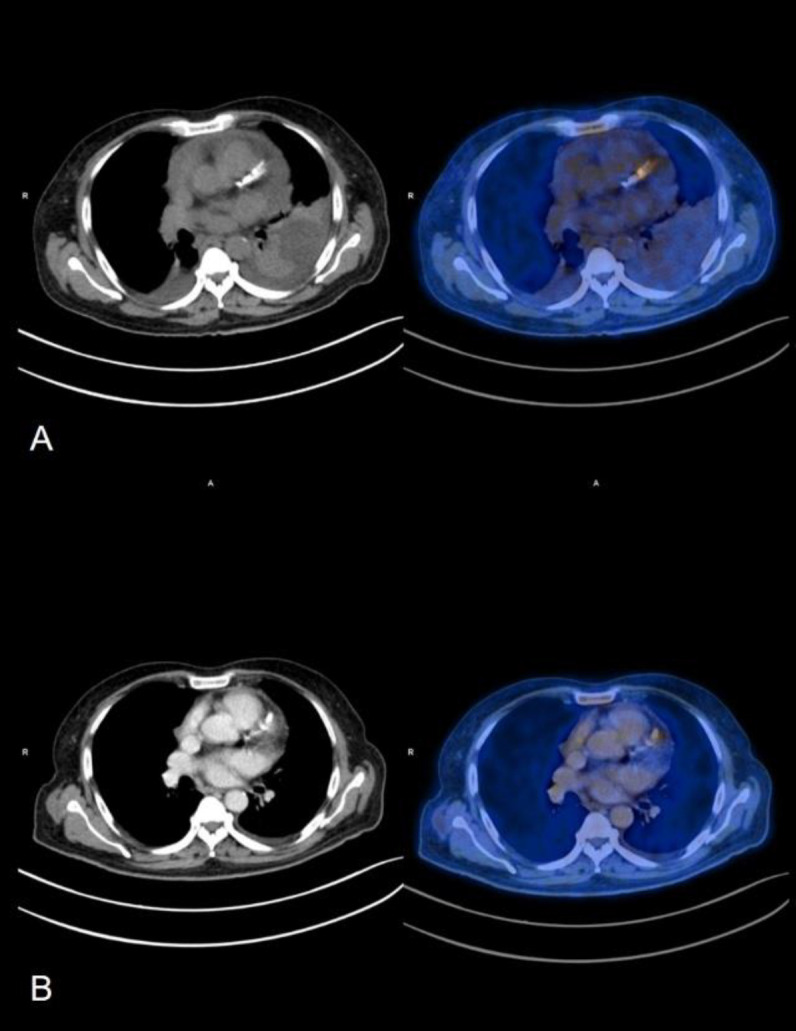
Transaxial CT and Fused FDG PET CT images (left to right); (A) showing abnormal increased FDG uptake (SUV_Max_: 3.5) along the LAD stent along with bilateral pleural effusion and mild pericardial effusion. Follow-up FDG PET CT (B) showing significant reduction in intenstiy & extent of FDG uptake along the LAD stent along with near complete resolution of pleural and pericardial effusion
